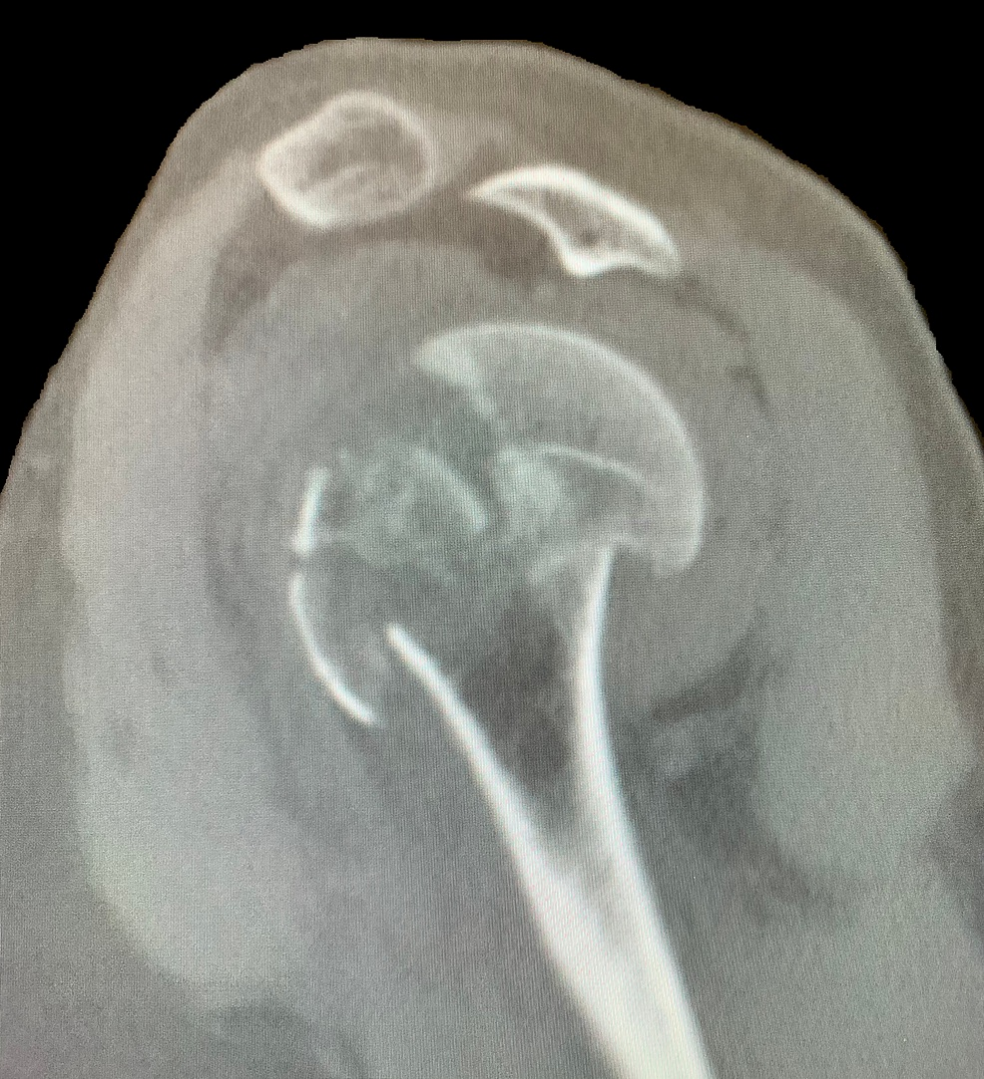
Sagittal view of preoperative CT scan of the left shoulder demonstrating a four-part proximal humerus (PH) posterior fracture-dislocation.
